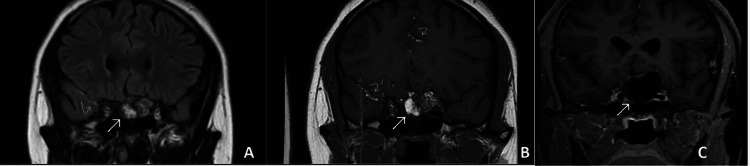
MRI coronal views of the dermoid cyst (straight arrow) and subarachnoid sulcal cholesterol deposits (curved arrows) on FLAIR (A), non-contrast T1 (B), and fat-suppressed non-contrast T1 (C) sequences. Notice the deposits disappear on fat-suppressed sequencing, consistent with their lipid nature.
